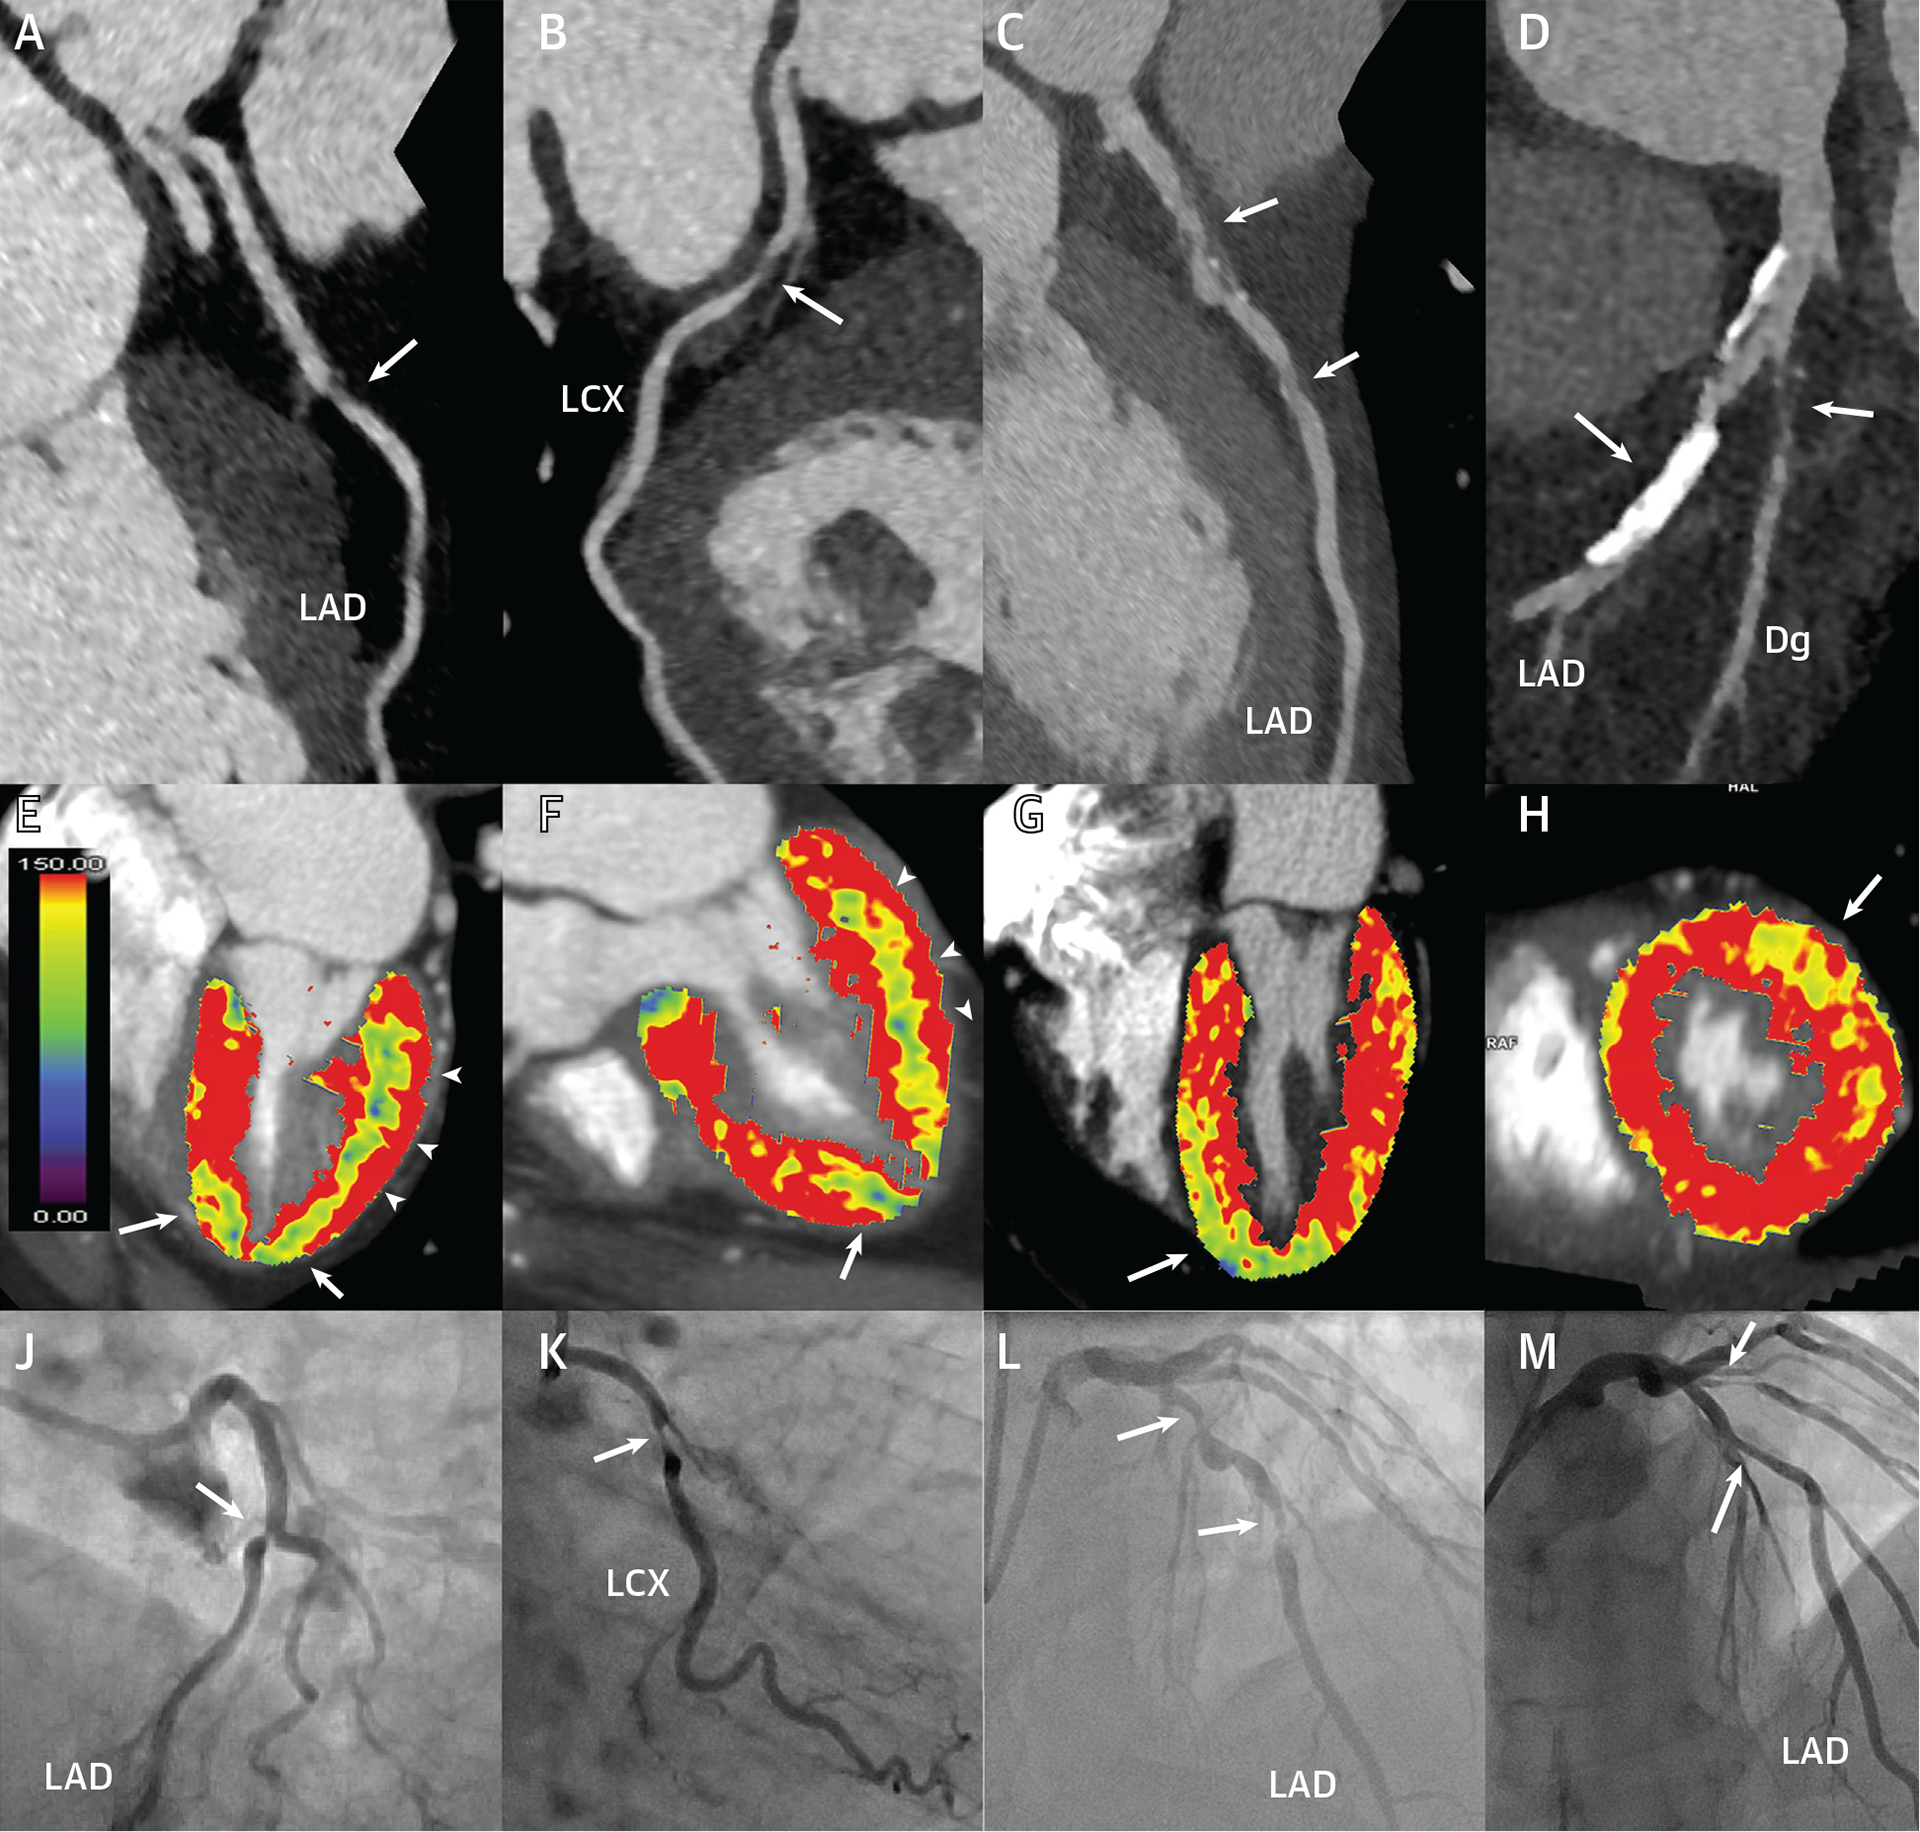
Case Examples Case 1: (A) Discrete narrowing in the LAD on CT (coronary CTA, arrows) and (E, F) an apical defect by perfusion imaging (CT-MPI, arrows) with (J) concordant ICA and an FFR of 0.76. The color bar in A displays the myocardial blood flow range from normal (red) to low (green and blue). (B) The same patient had a second stenosis in the LCX, with (E, F) a posterolateral perfusion defect (arrowheads), concordant with (K) ICA and FFR of 0.74. Case 2: (C) Diffuse, partially calcified narrowing and focal dilatation in the LAD on coronary CTA and a (G) CT-MPI perfusion defect in the distal septum and apex, confirmed by (L) ICA and an FFR of 0.56. Case 3: (D) Coronary CTA shows severely calcified plaque of uncertain angiographic stenosis severity in the LAD and a predominantly noncalcified severe stenosis in a large Dg. (H) There is a distinct anterolateral perfusion defect subtended by the Dg (arrow) but normal blood flow in the LAD territory. (M) ICA confirms the severe Dg stenosis (FFR: 0.68) and functionally nonsignificant, moderate mid-LAD stenosis (FFR: 0.83). CT = computed tomography; CT-MPI = computed tomography myocardial perfusion imaging; CTA = computed tomography angiography; Dg = diagonal branch; FFR = fractional flow reserve; ICA = invasive coronary angiography; LAD = left anterior descending coronary artery; LCX = left circumflex coronary artery.
